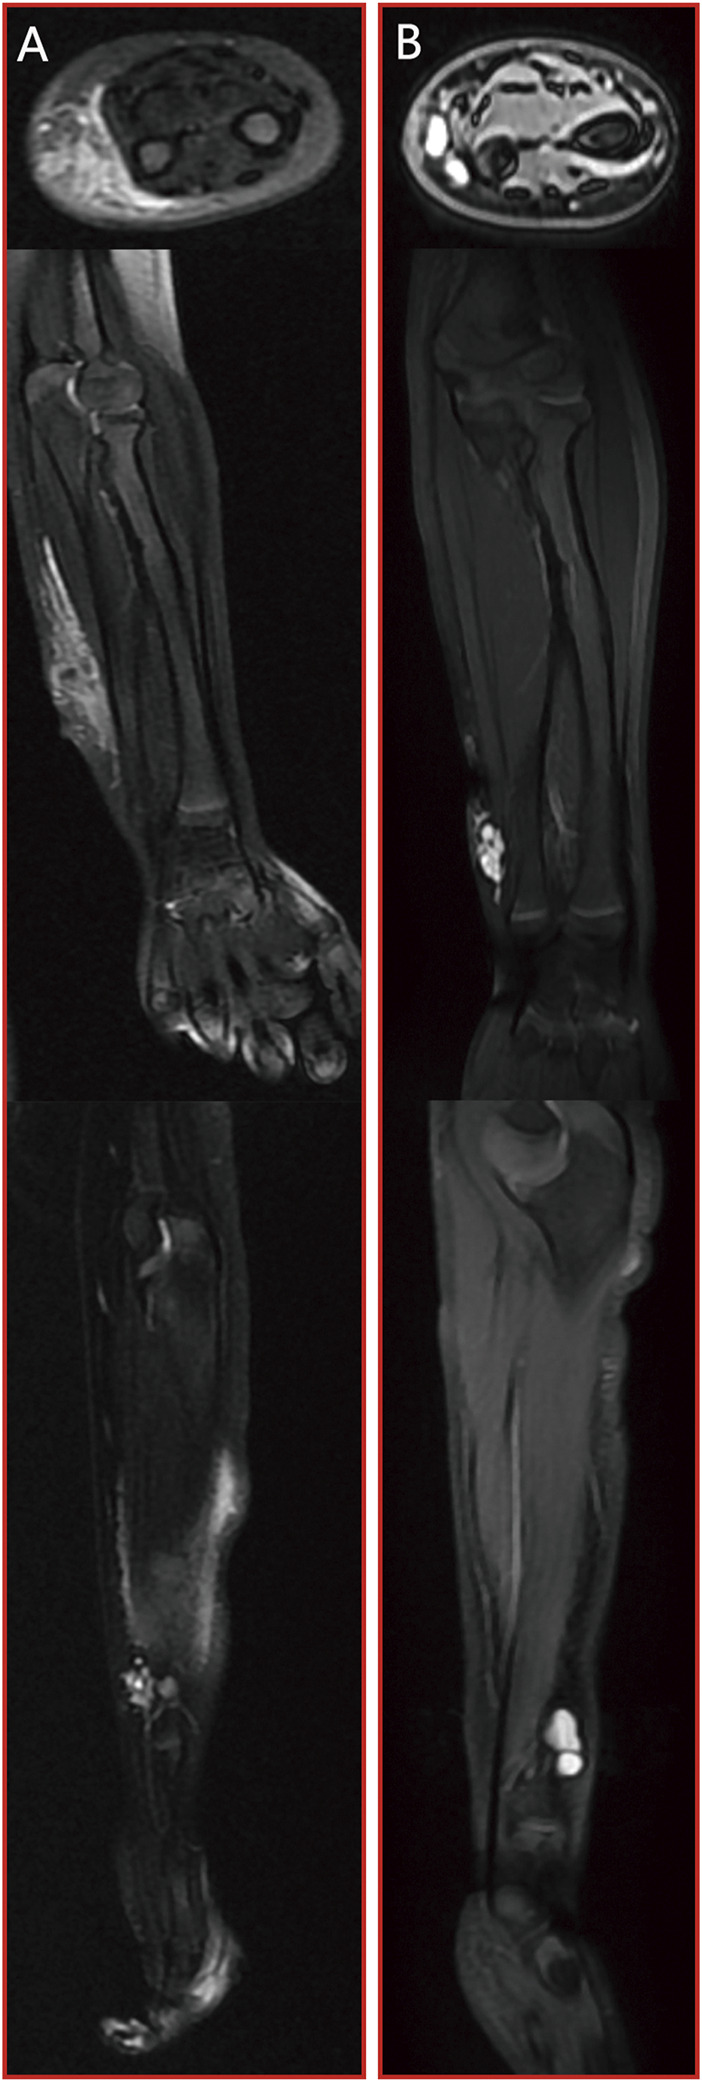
Case 4: patient with microcystic LMs of left forearm which achieved a significant and remarkable improvement after three sessions of combination treatment. (A) pre-treatment MRI stud; (B) post-treatment MRI study. From top to bottom: axial T2 fat saturated image, coronal T2 fat saturated images and sagittal T2 fat saturated images.
